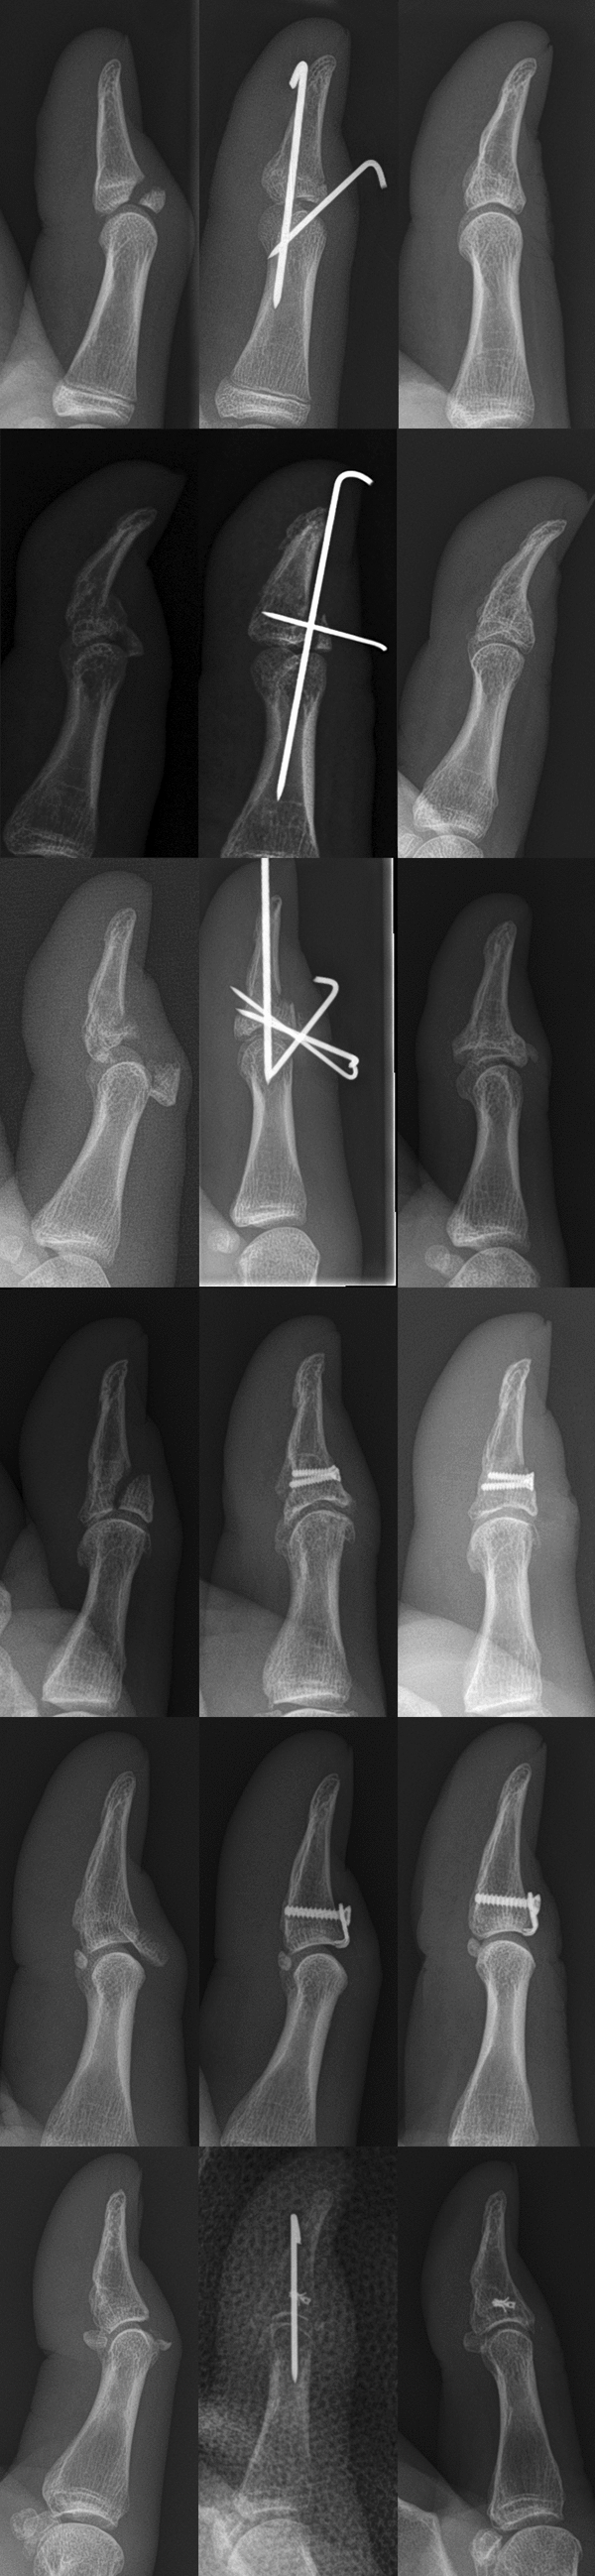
Radiographs of different treatment methods of a bony mallet thumb fracture (after injury, after surgery and at final follow-up)
